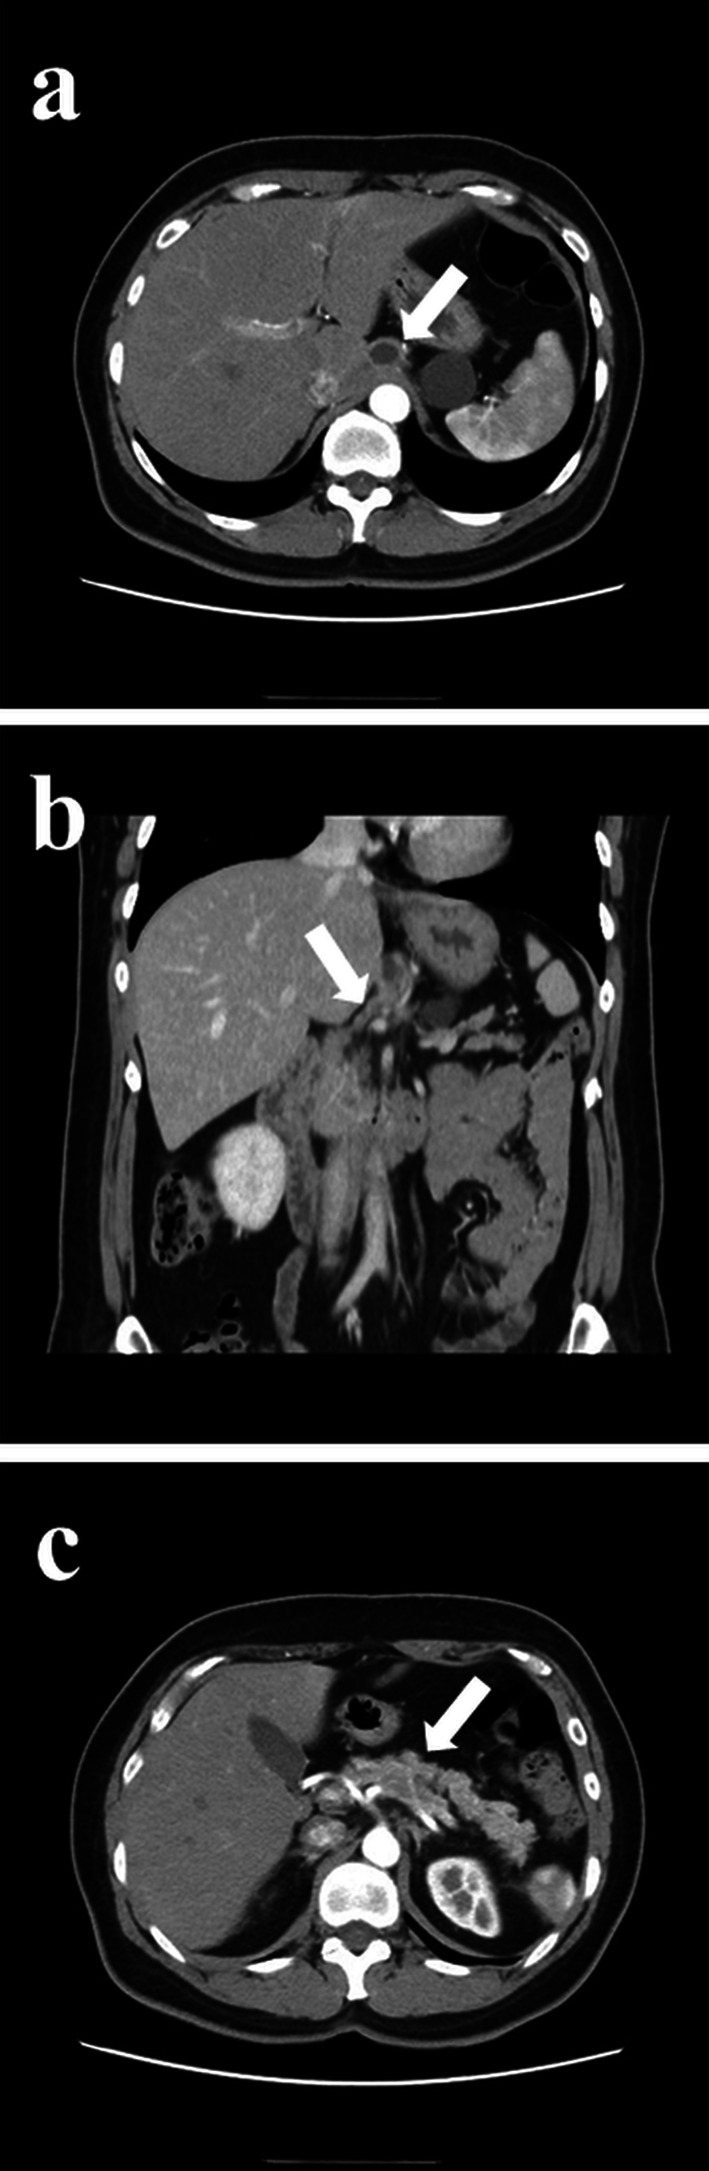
CT images before neoadjuvant chemotherapy. a A cystic nodular lesion observed beside the crus (white arrow), and cystic formation of PD was observed. b The nodular lesion was the GDC and connected to the pancreas (white arrow). c A 16-mm mass with poor contrast effect on the pancreatic body was detected (white arrow)
